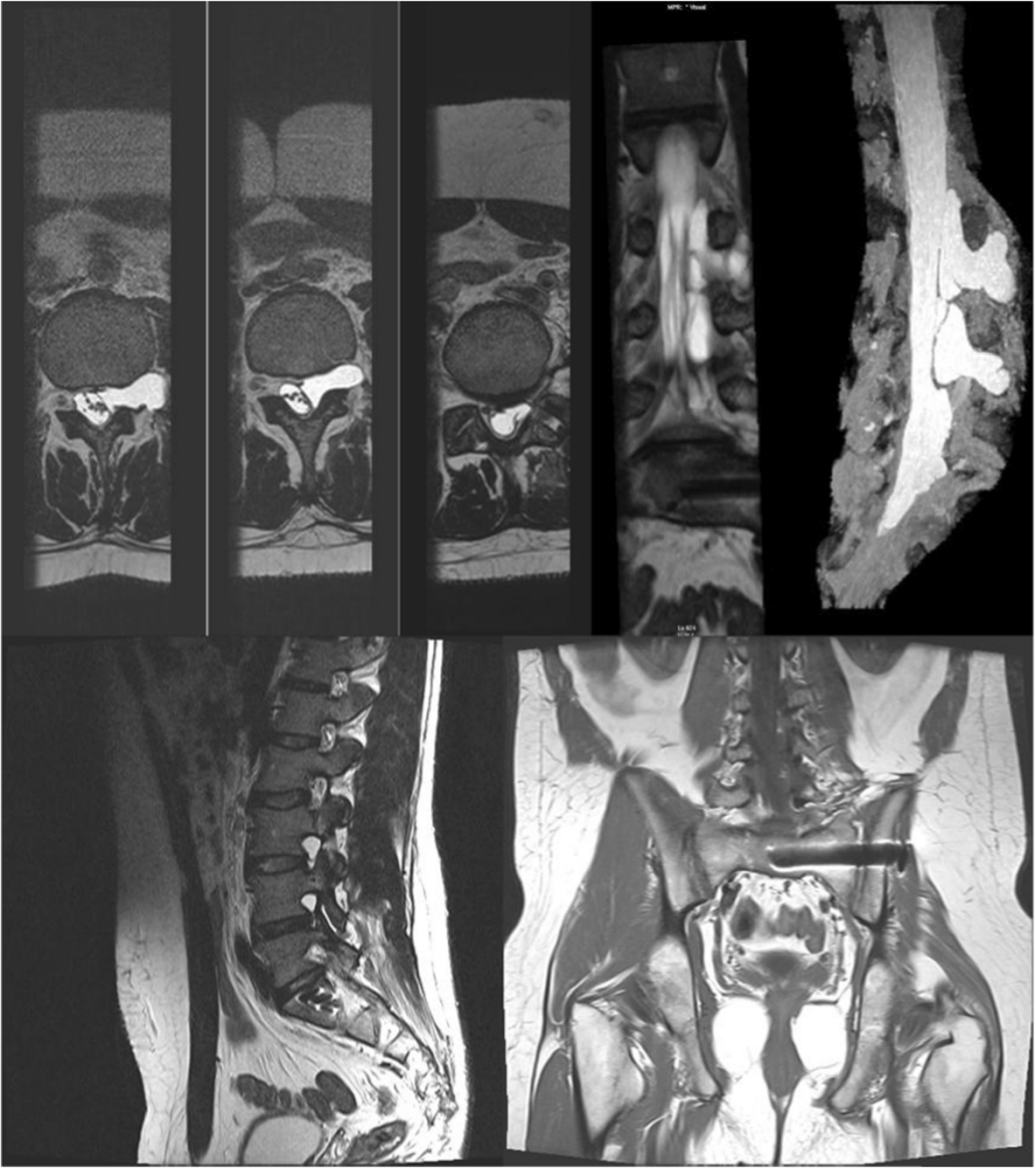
MRI study of complete LSP injury with multiple avulsions.
